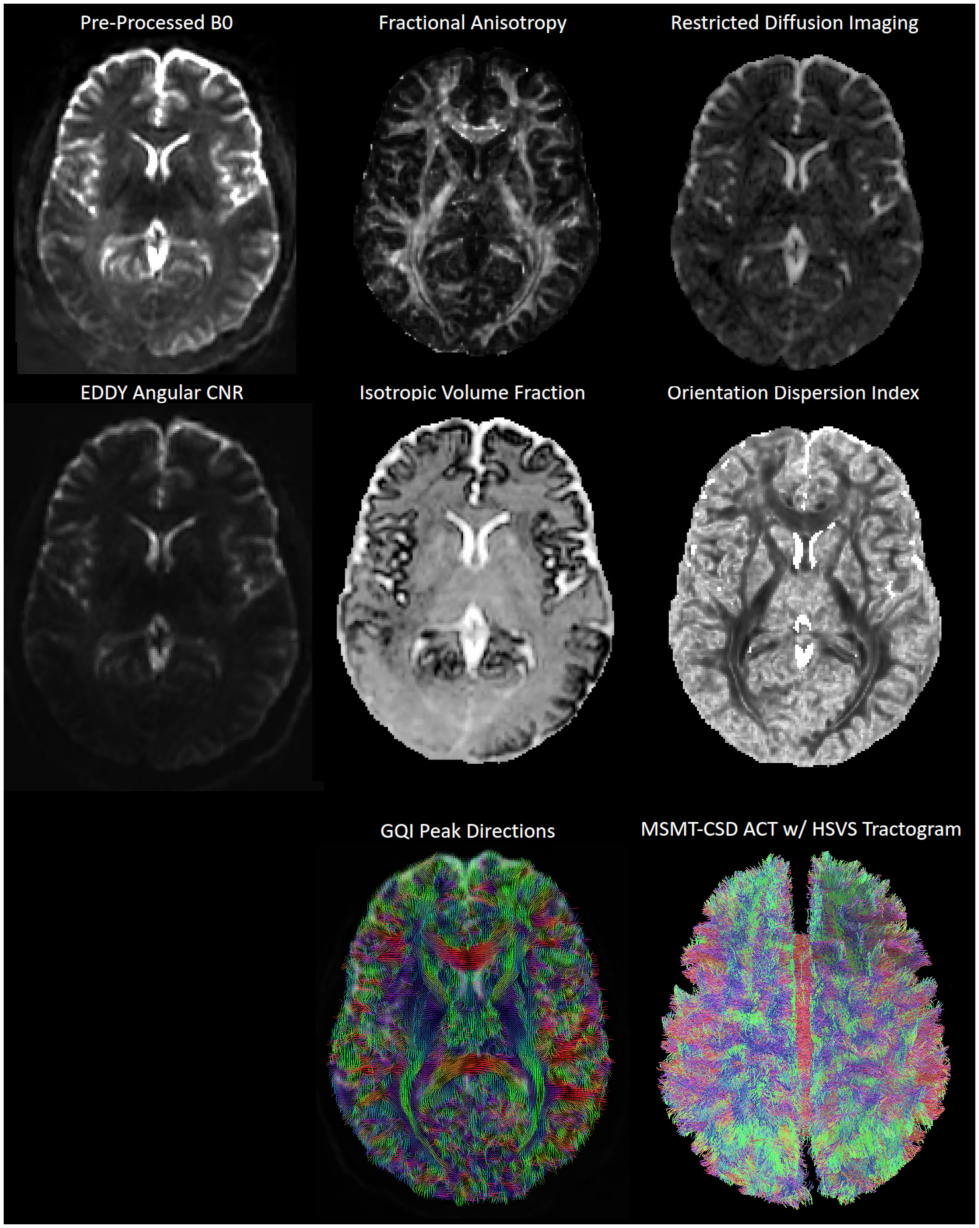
Example diffusion MRI data and outputs from one participant in CUPS. B0: *b*-value 0, CNR: contrast-to-noise ratio (from FSL EDDY), GQI: generalized *q*-sampling imaging, MSMT-CSD ACT w/ HSVS: multi-shell multi-tissue constrained spherical deconvolution reconstructed anatomically constrained tractography with hybrid surface-volume segmentation.
